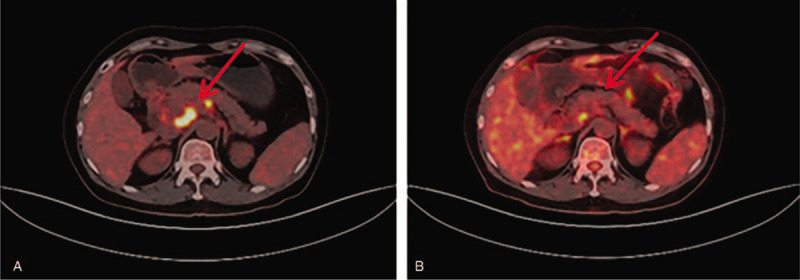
PET-CT showed multiple lymph node metastases in the retroperitoneum. (A) Before treatment with pembrolizumab and nab-paclitaxel, the largest lymph node was 1.6 cm in short diameter. (B) After 6 cycles of pembrolizumab and nab-paclitaxel, the metastases were mostly dismissed. PET-CT = positron emission tomography-computed tomography.
